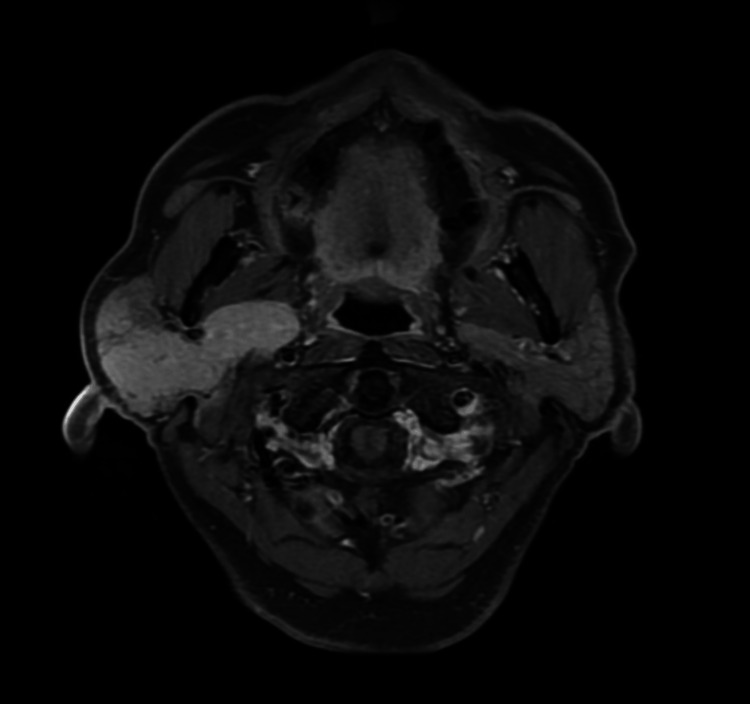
T1 post-contrast fat-suppressed axial MRI demonstrating an ill-defined, hyperintense mass in the right parotid gland.
